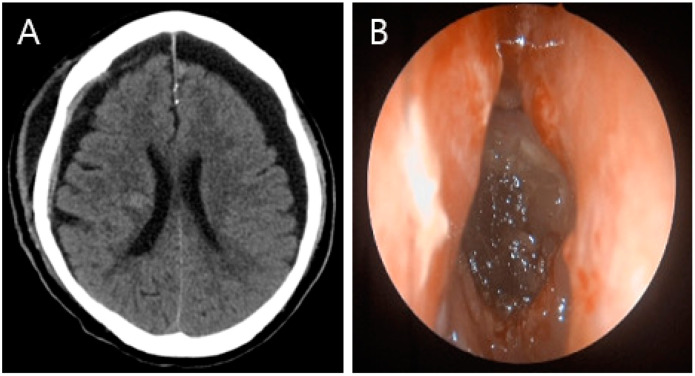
One-month follow-up. (A) Brain CT image, showing no specific findings except for bilateral hygroma. (B) Nasal endoscopy, showing that the defective area healed well without any specific findings.
